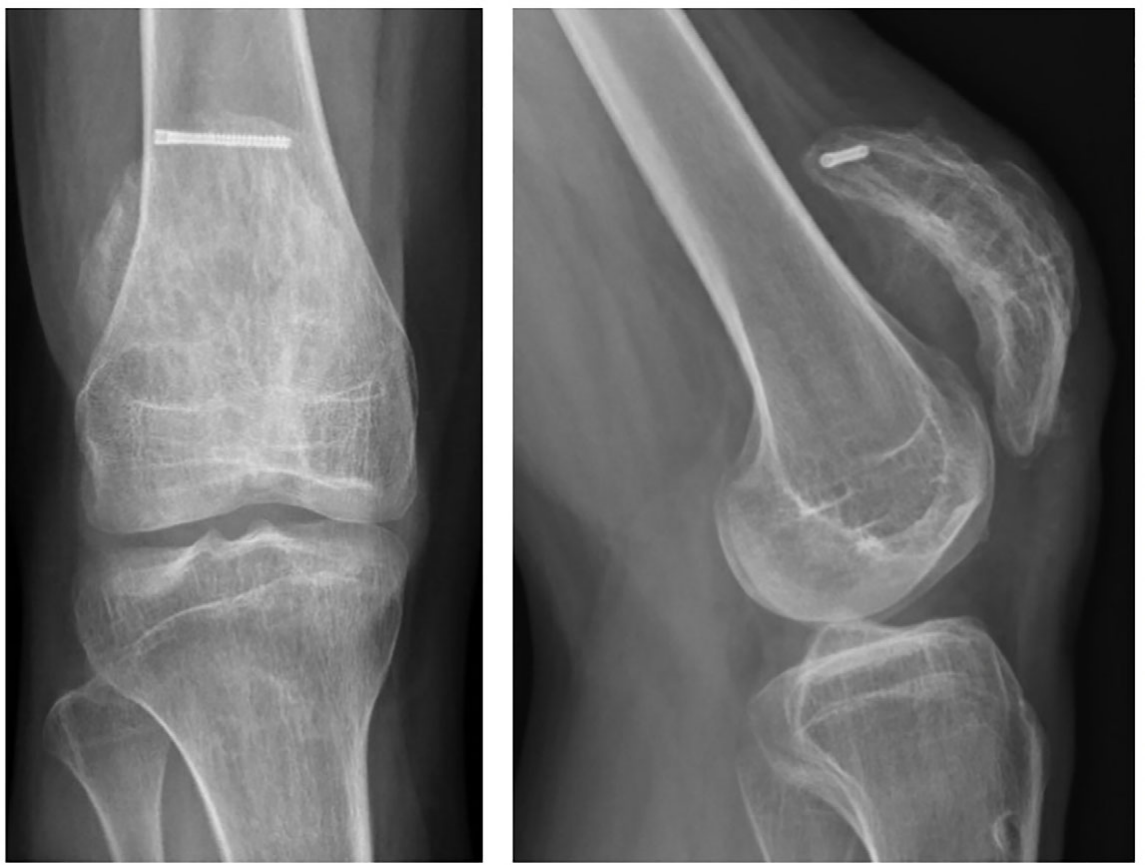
AP and lateral radiographs after hardware removal.
